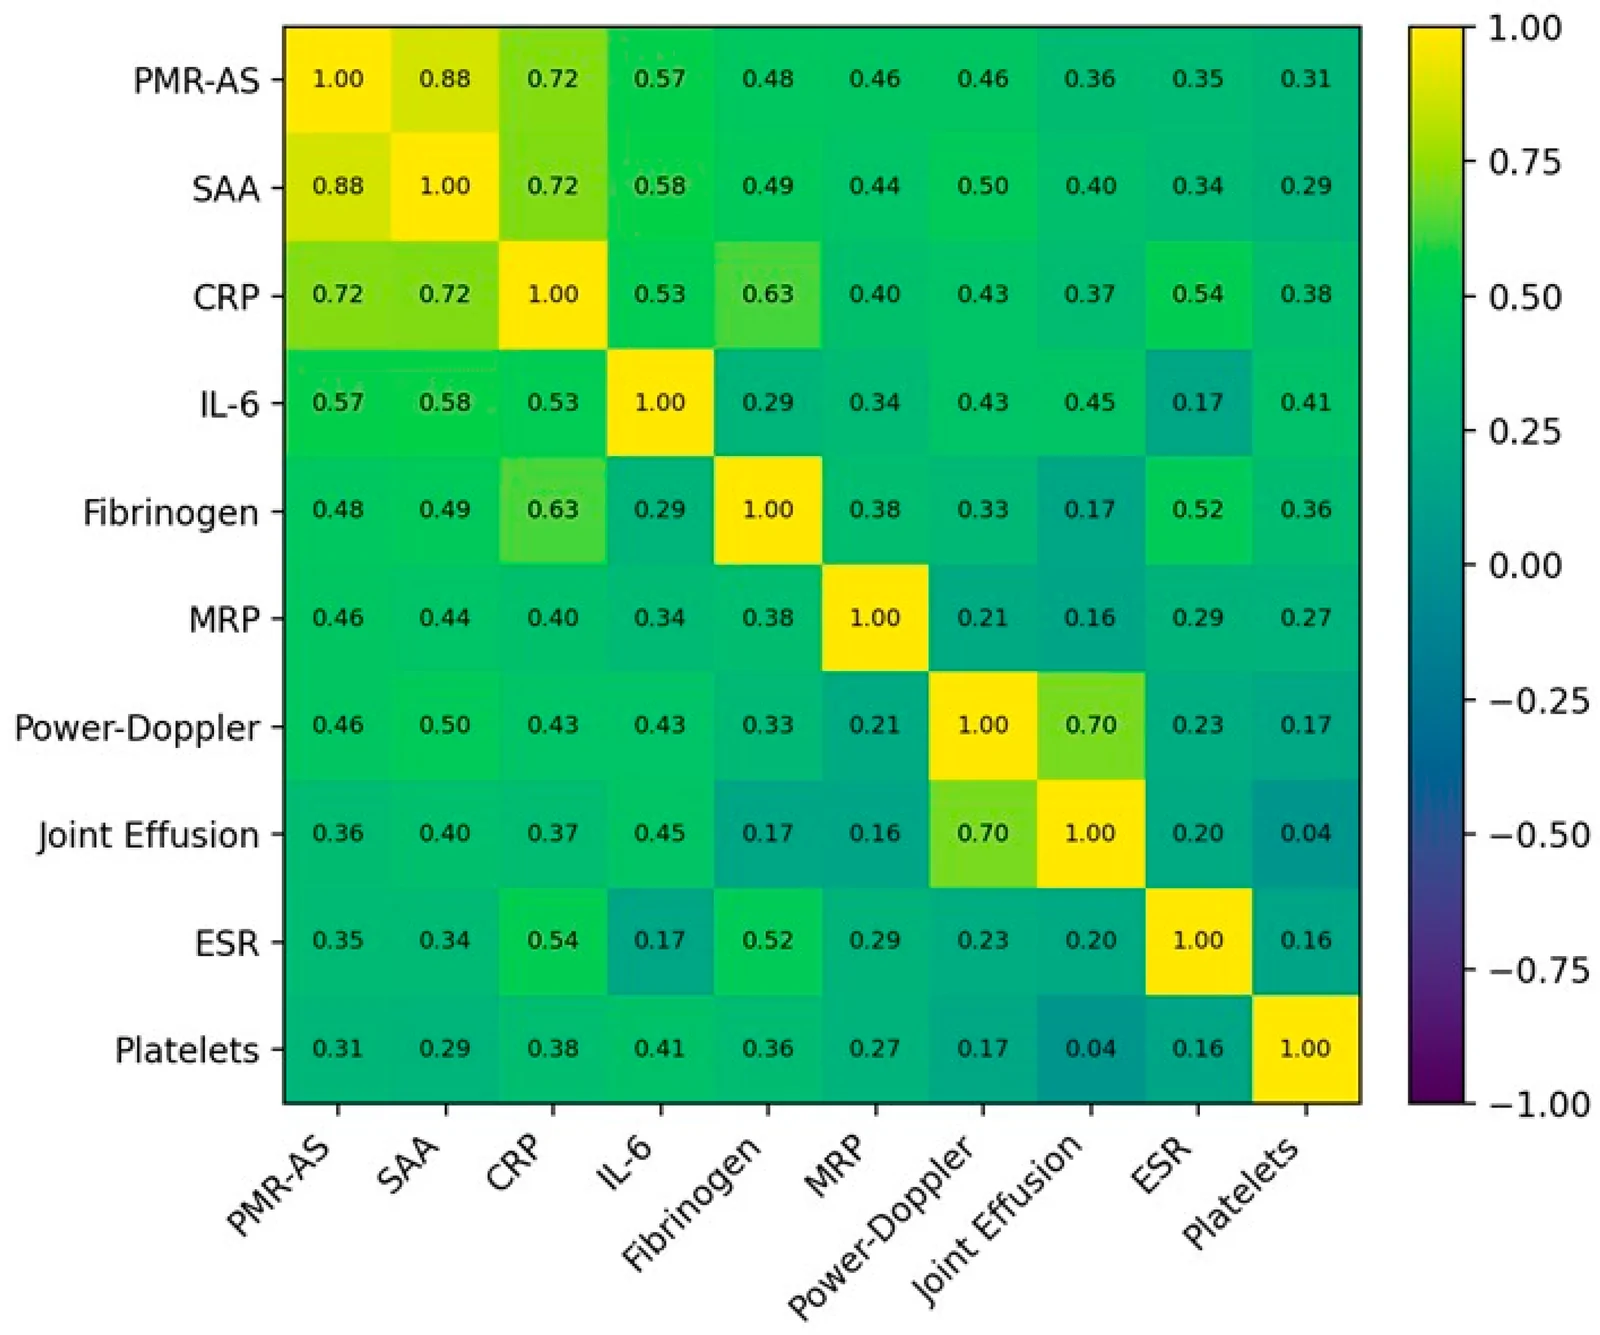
Spearman correlation heatmap among PMR-AS and key inflammatory variables. Darker positive values indicate stronger direct associations. SAA shows the strongest correlation with PMR-AS, followed by CRP, IL-6, fibrinogen, MRP, and Power-Doppler signal.
